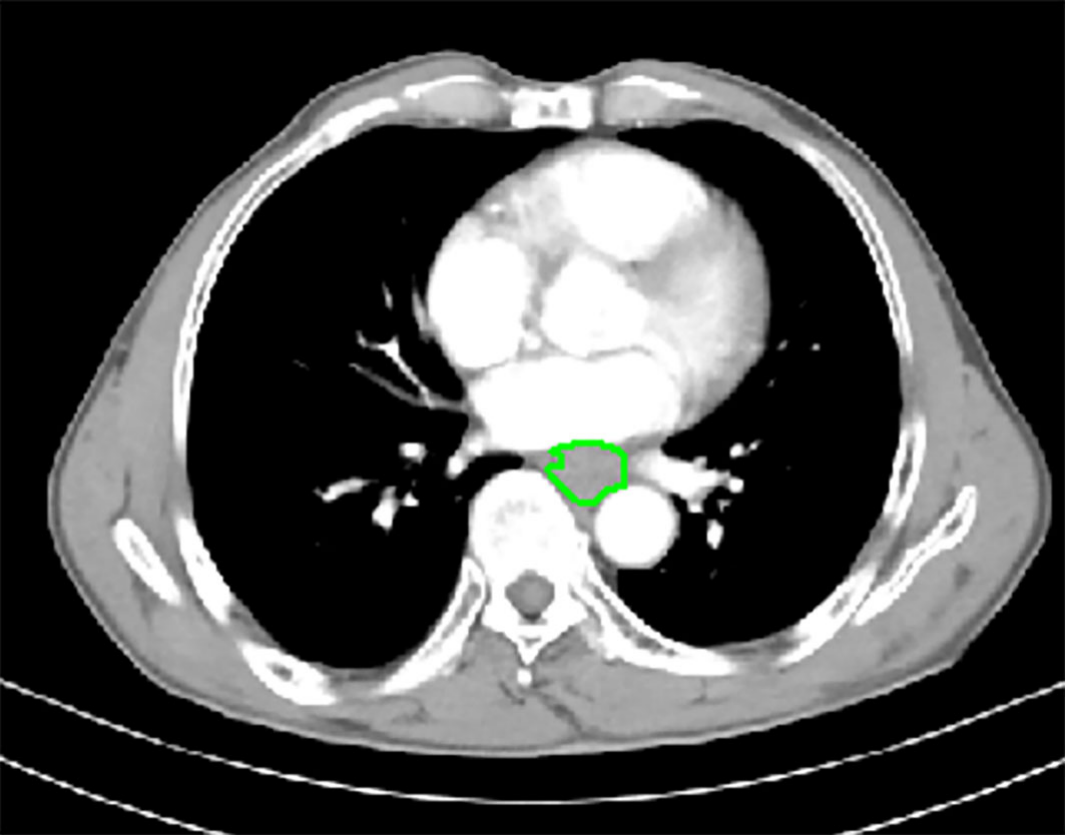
In a 66-year-old male with esophageal squamous cell carcinoma at cT_3_N_0_M_0_, the preoperative thoracic contrast-enhanced CT scans depict the gross tumor volume obtained by manual delineation along the margin of the abnormal esophageal wall slice-by-slice, and the gross tumor volume is 12.83 cm^3^. During the follow-up period, there was no recurrence as shown on follow-up CT after radical esophagectomy.
